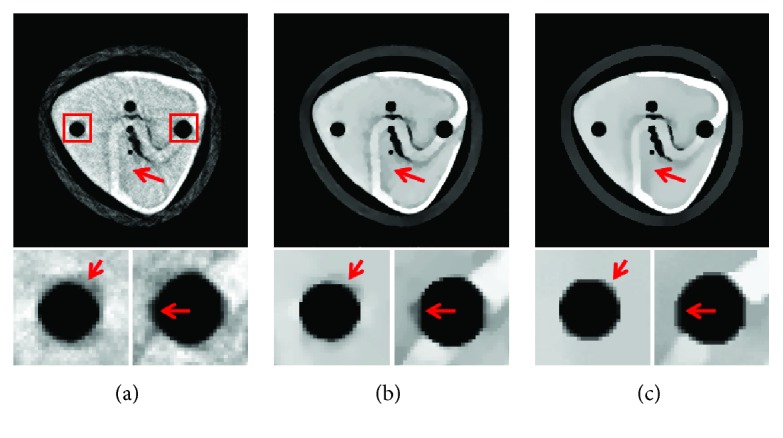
CT images of the modified buckwheat seed in experiment 2: (a) SART, (b) TV, and (c) TF-L0. The second row images are the close-ups of ROIs. The places pointed out by red arrows are examples where TF-L0 acquires the best results.
